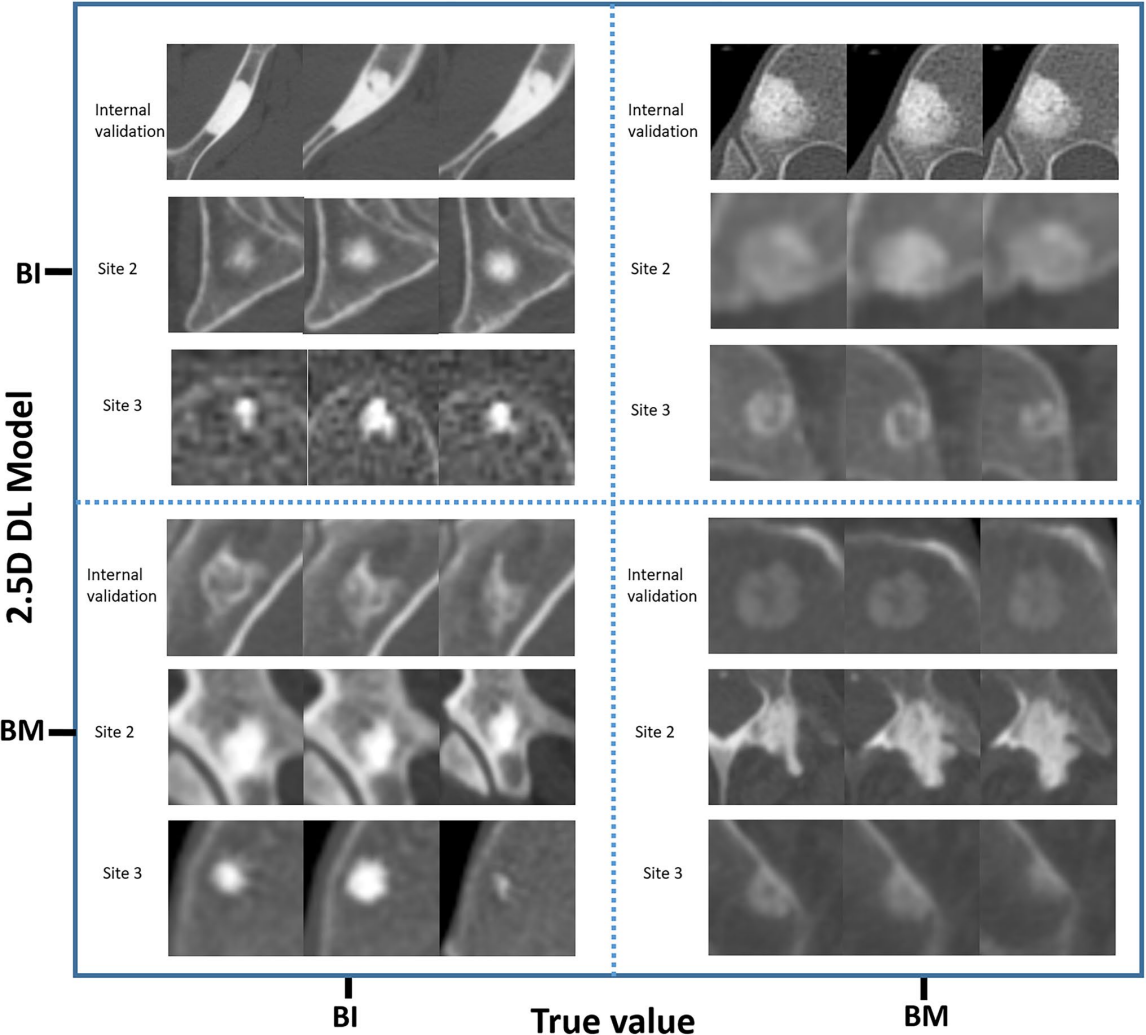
Examples of the 2.5D DL model evaluating correct and incorrect CT images in the internal validation set, in the external validation set from site 2, and in the external validation set from site 3
